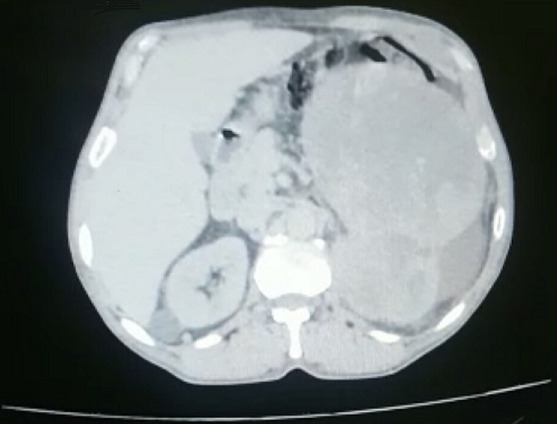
CT scan showing left renal tumor
